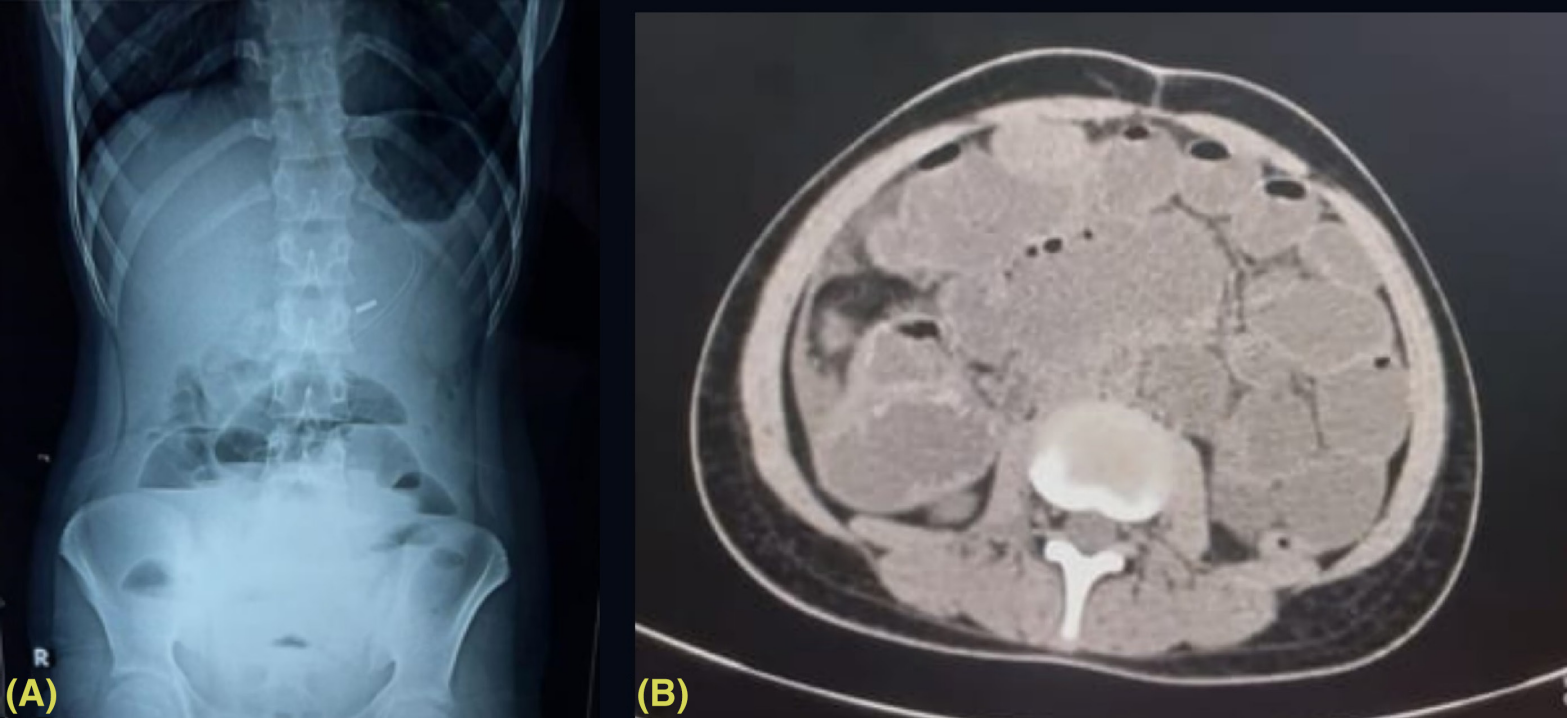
(A) Chest X‐ray of patient. (B) Computed tomography scan of the abdomen showing increased wall thickness near the hepatic flexure.
